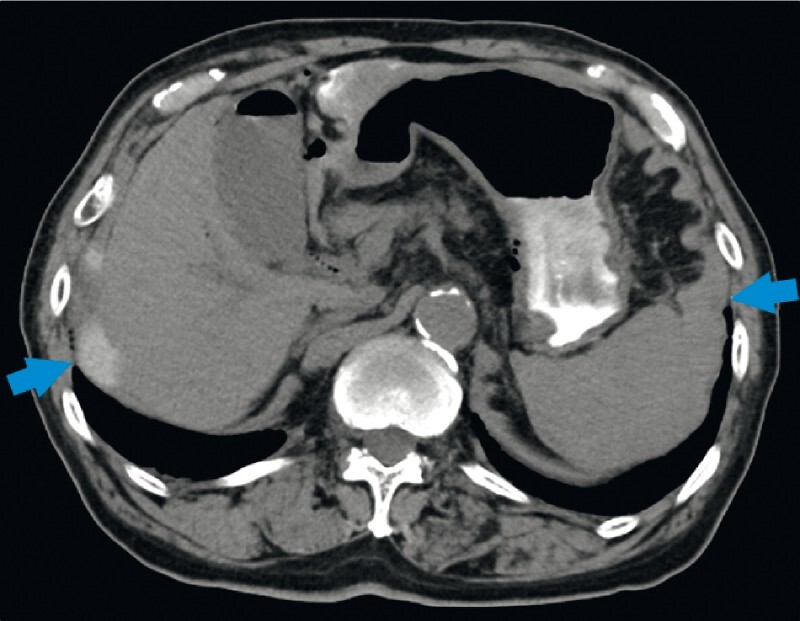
Postprocedure axial computed tomography showing hematomas around the liver and spleen (arrowheads).
